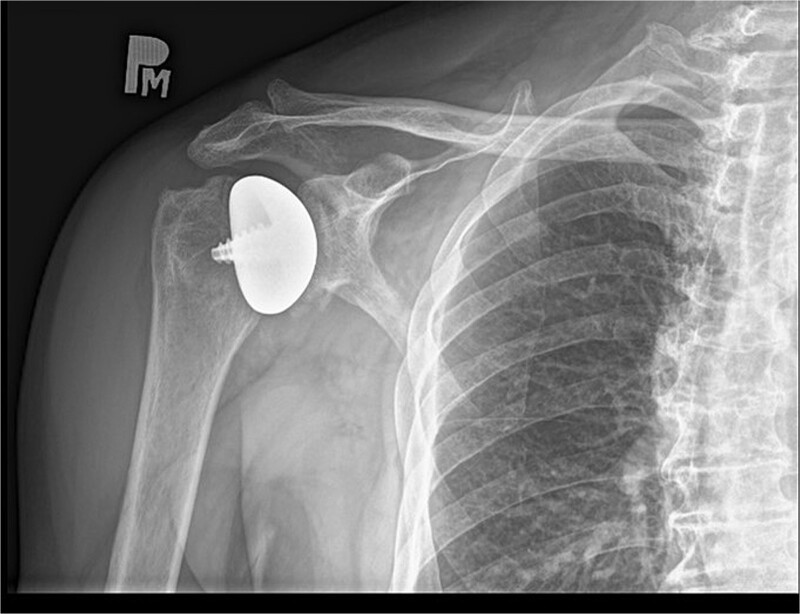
X-ray image after 16 days from the surgery.
